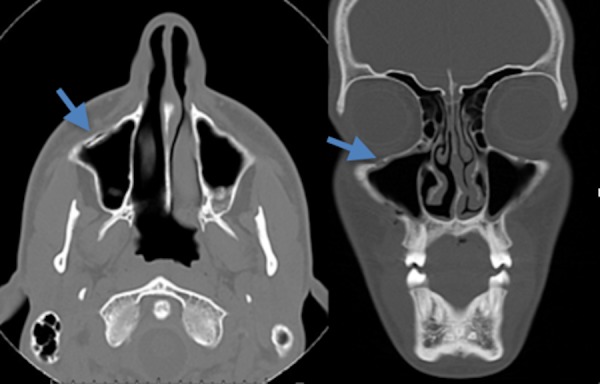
Minimally displaced maxillary sinus wall fracture without sinus opacification Axial (left) and coronal (right) CT scan of the facial bones demonstrates a non-displaced fracture (blue arrow) involving the anterior inferior wall of the right maxillary sinus. There is no associated fluid seen within the sinus.
